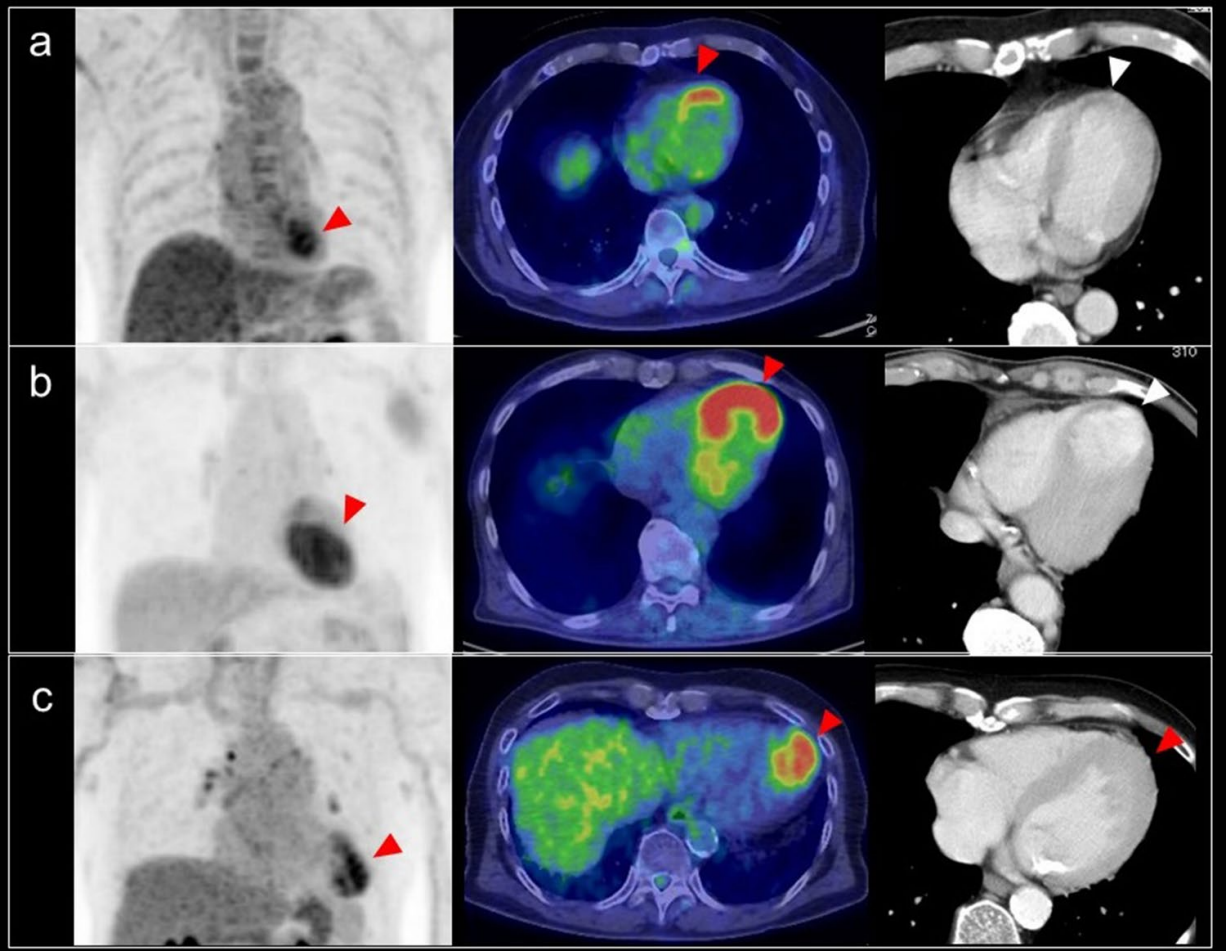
Focal FDG uptake in the left ventricular apex. a focal FDG uptake in the left ventricular (LV) apex (red arrowheads) matched the liner unenhanced CT area suggesting chronic ischemic change of the apex (white arrowhead). b intense focal FDG uptake in the LV apex (red arrowheads) was matched to the LV aneurysm (white arrowhead). c apical hypertrophic cardiomyopathy can also show focal FDG uptake in the LV apex (red arrowheads)
